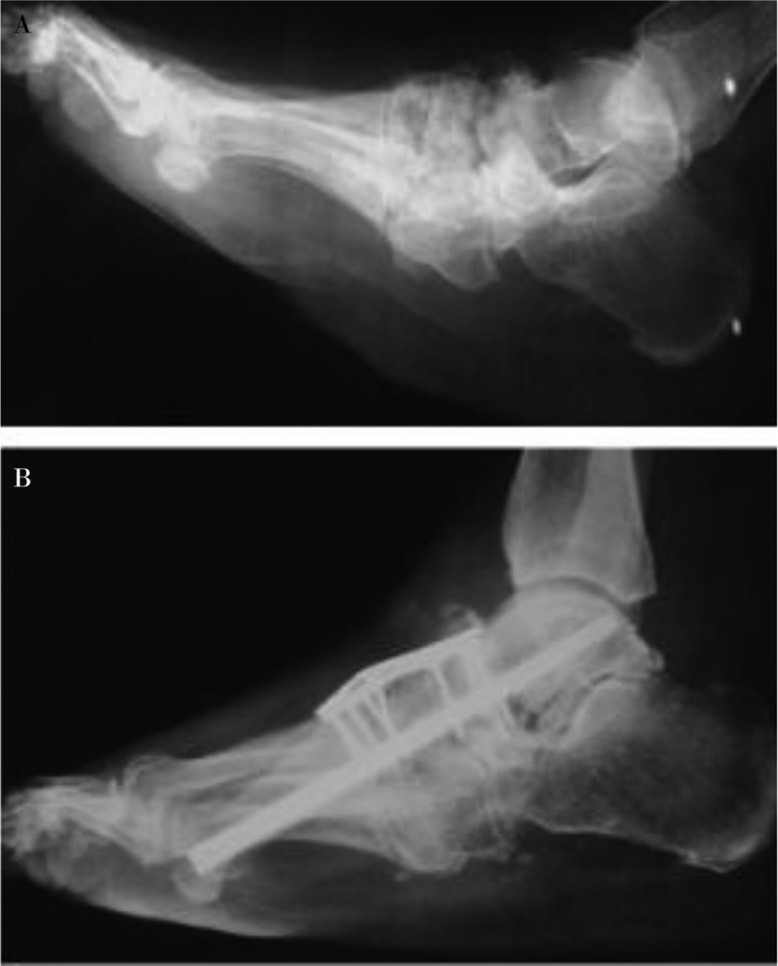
A 62-year-old female with a 10-year history of diabetes mellitus and Stage III chronic Charcot neuroarthropathy. Preoperative lateral radiograph (a) and postoperative lateral radiograph (b) showing the arthrodesis with internal fixation after osteotomy and deformity correction.
